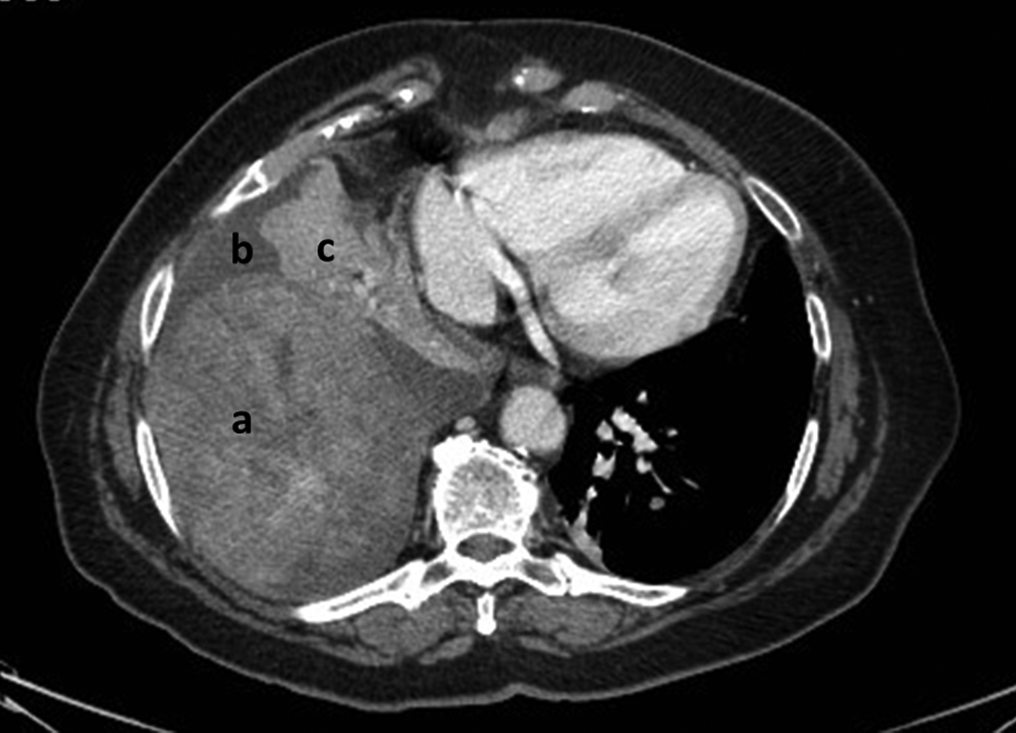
Initial computed tomography (CT) scan showing: a, large rounded pulmonary benign leiomyoma; b, pleural effusion; c, passive lung atelectasis.
